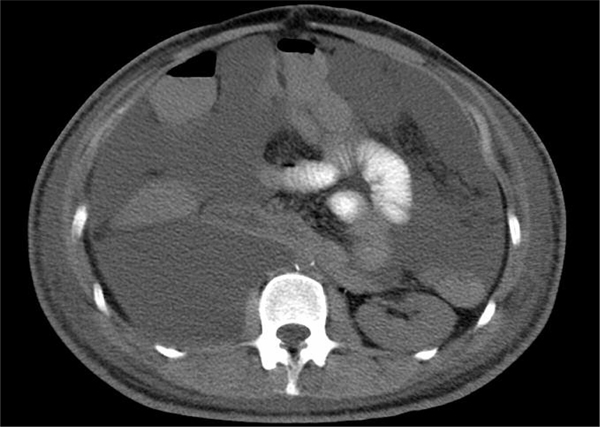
A CT scan of the abdomen with an oral contrast agent, demonstrating marked intra-abdominal lymph leakage postoperatively with organ compression and abdominal compartment syndrome.
